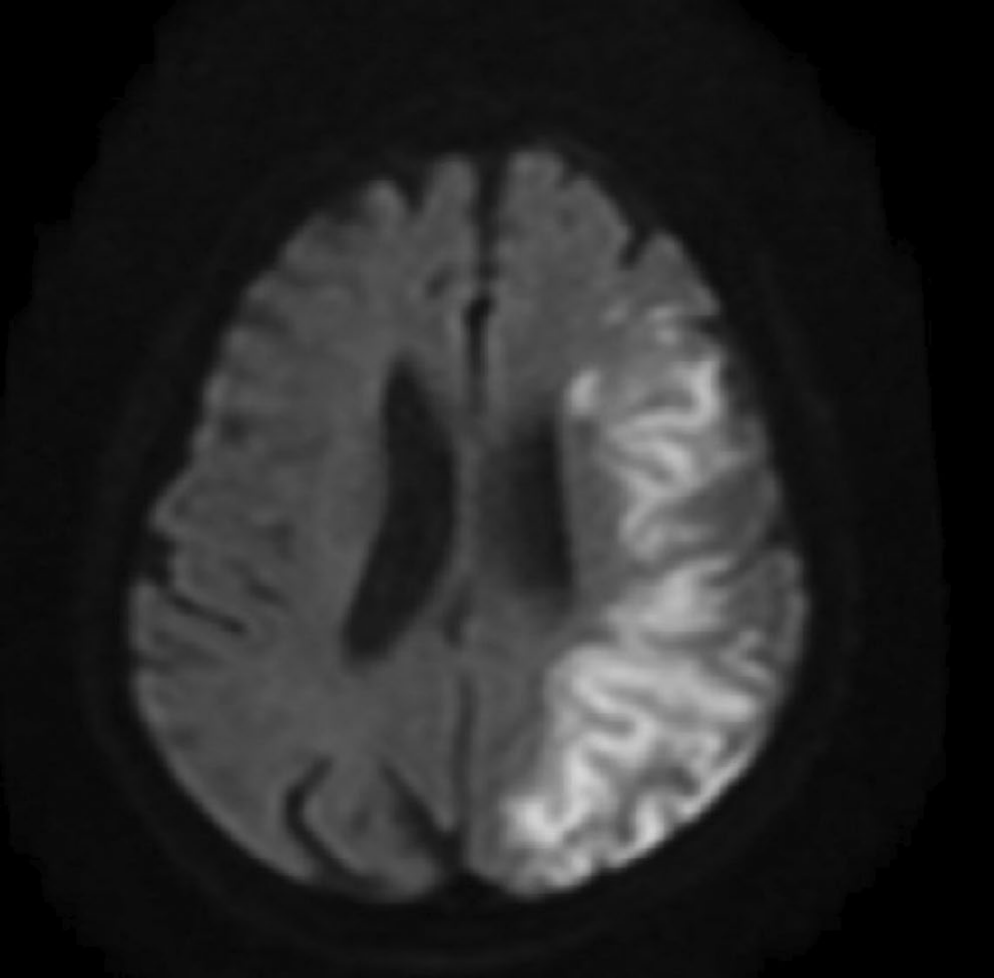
FIGURE 2 MRI axial DWI image
